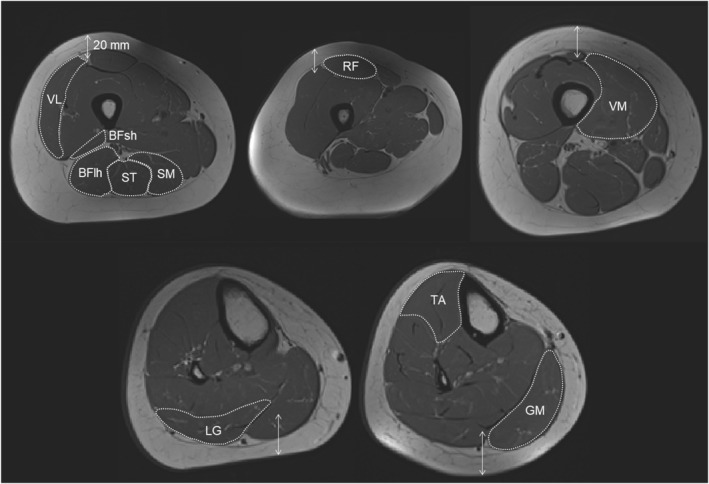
Representative MR images (female participant). BFlh, biceps femoris long head; BFsh, biceps femoris short head; GL, gastrocnemius lateralis; GM, gastrocnemius medialis; RF, rectus femoris; SM, semimembranosus; ST, semitendinosus; TA, tibialis anterior; VL, vastus lateralis; VM, vastus medialis.
